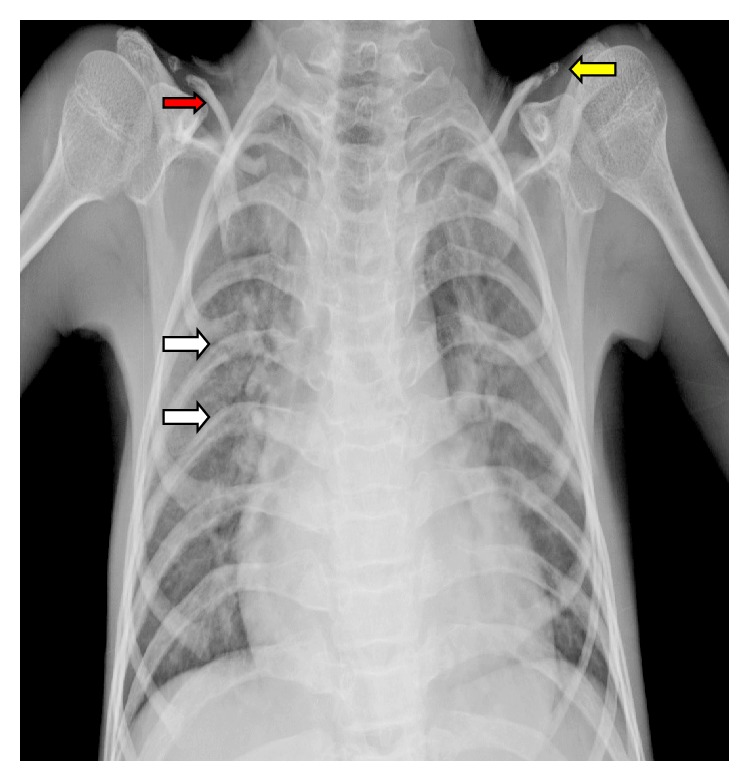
Frontal chest radiograph showing cardiomegaly, sloping slender fifth and sixth posterior ribs (white arrows). The absence of lateral and medial end of both clavicles (red arrow) with a tiny bone fragment was seen in the acromial end of both clavicles (yellow arrow).
